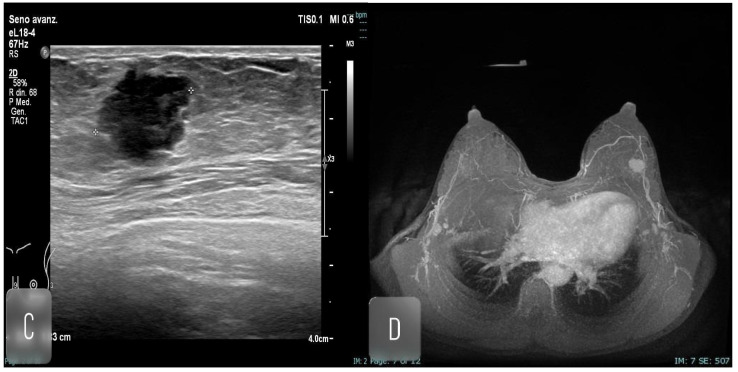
Forty-one yo. breastfeeding woman, with palpable lump in left breast. Mammography detected a radiopacity (20 mm). Core needle biopsy reveals high grade apocrine invasive carcinoma. (A,B) MLO and CC mammography projections; (C) US imaging; (D) MRI, MIP.
